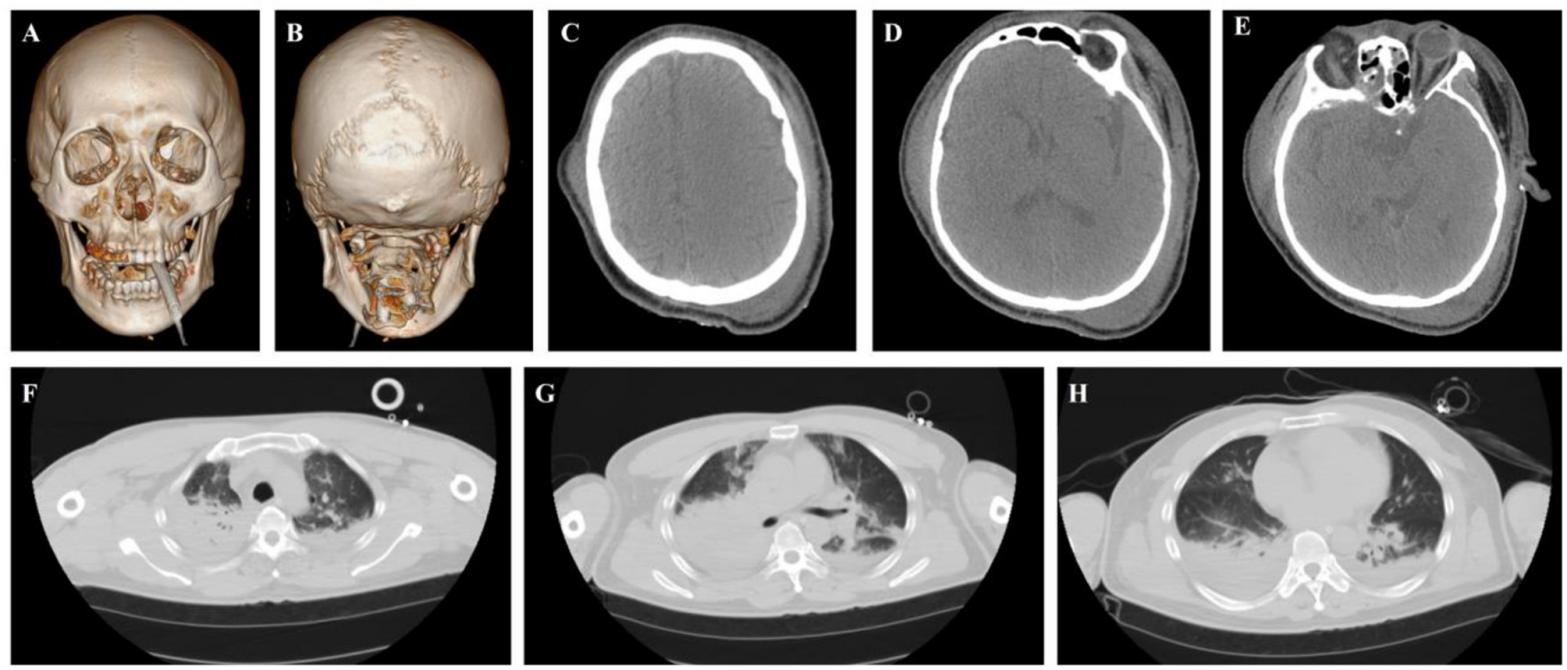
Critical Patient 8: cranial and thoracic imaging examinations. (A–E) CT of the head reveals mild cerebral edema. (F–H) Chest CT shows bilateral pulmonary infiltrates with consolidation, along with contusion.
